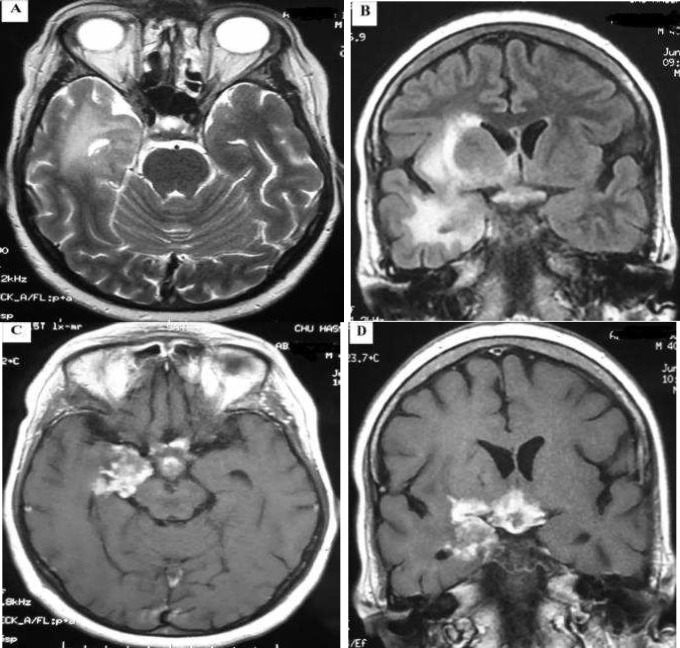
Cerebral magnetic resonance imaging (MRI) showing hyperintensities in the right temporoinsular region associated with an enhancement and nodular leptomeningeal thickening of the basilar peri-mesencephalic citern extended to the right temporal lobe, hypothalamus and walls of the third ventricle. (A) T2-weighted images, (B) FLAIR sequences, (C and D) gadolinium enhanced T1-weigthed images
